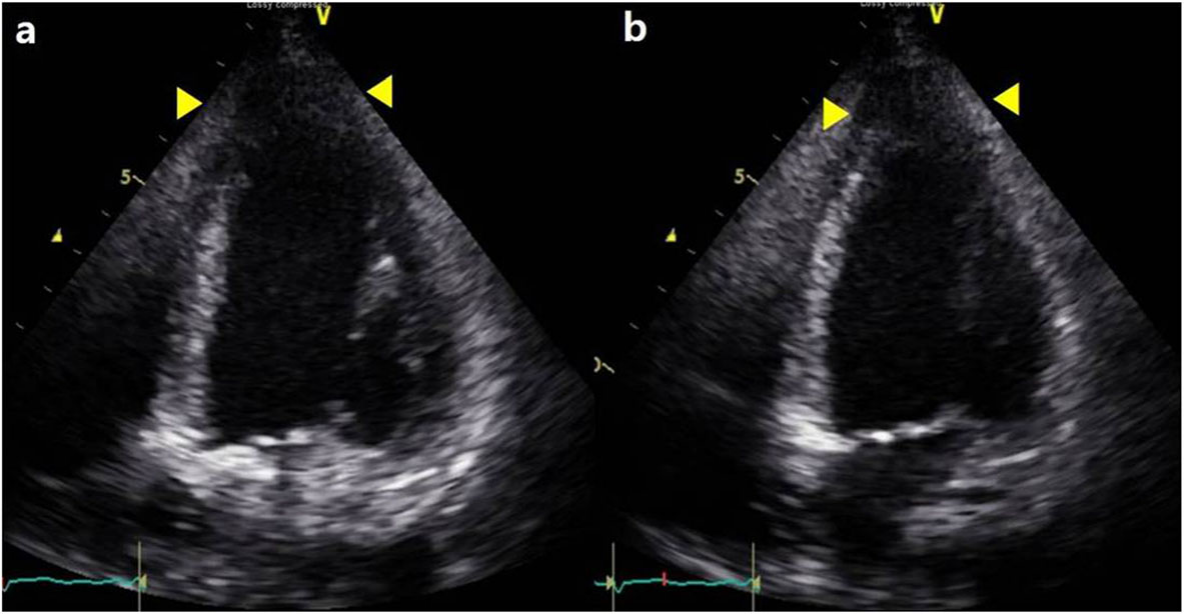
a, b Thoracic echocardiography showing akinesia on the base to the mid left ventricle (left, end-diastole; right, end-systole)
